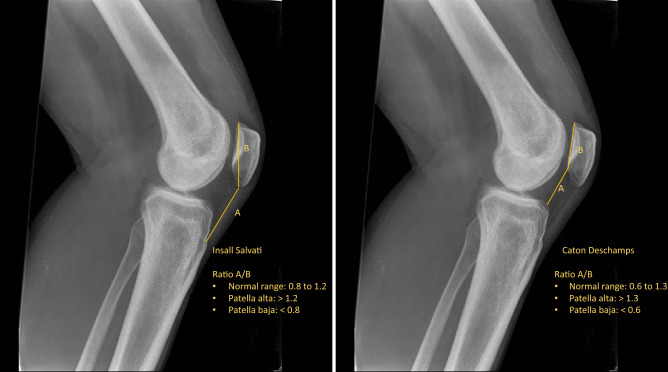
Methods to evaluate the IS and CD on plain radiographs
